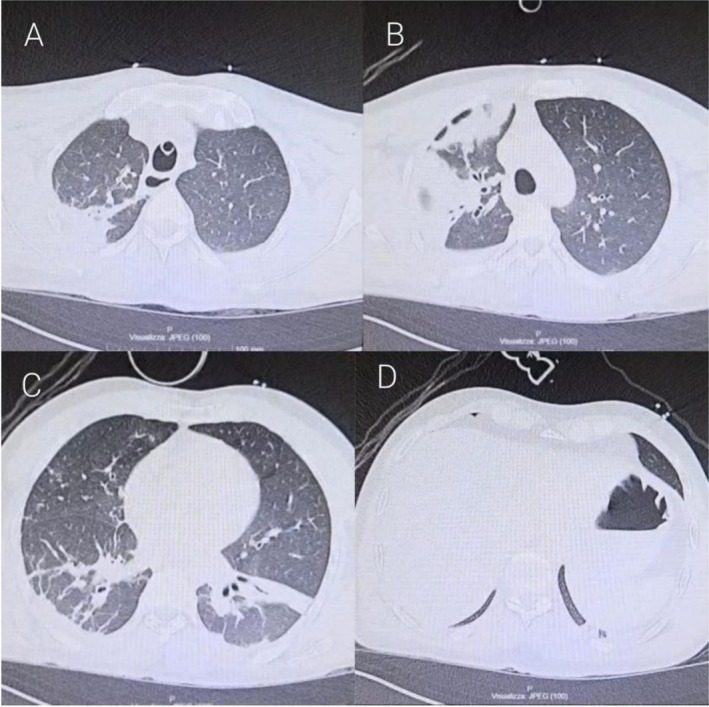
Series of chest CT scan slices demonstrating bronchioloalveolar distribution consolidations in the middle lobe. Additional consolidation areas with hypoventilatory appearance are visible: (A) in the right upper lobe, predominantly peripherally and along the fissures; (B) peripherally in the right lower lobe; (C) more limited, in the fissural and basal segments of the left upper lobe. (D) A more caudal chest CT slice showing a markedly distended gastric lumen with an air–fluid level at the fundus.
